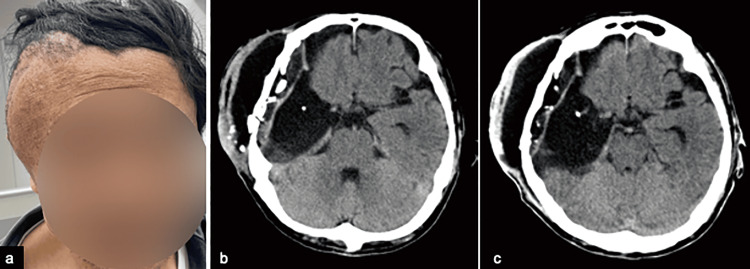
Pseudomeningocele at the surgical site (a)The patient presented pseudomeningocele that gradually worsened two weeks after tumor resection. (b) CT showed intractable subcutaneous effusion that gradually worsened in weeks after the primary surgery. (c) Pseudomeningocele was not resolved despite the placement of lumbar drainage for two weeks.
